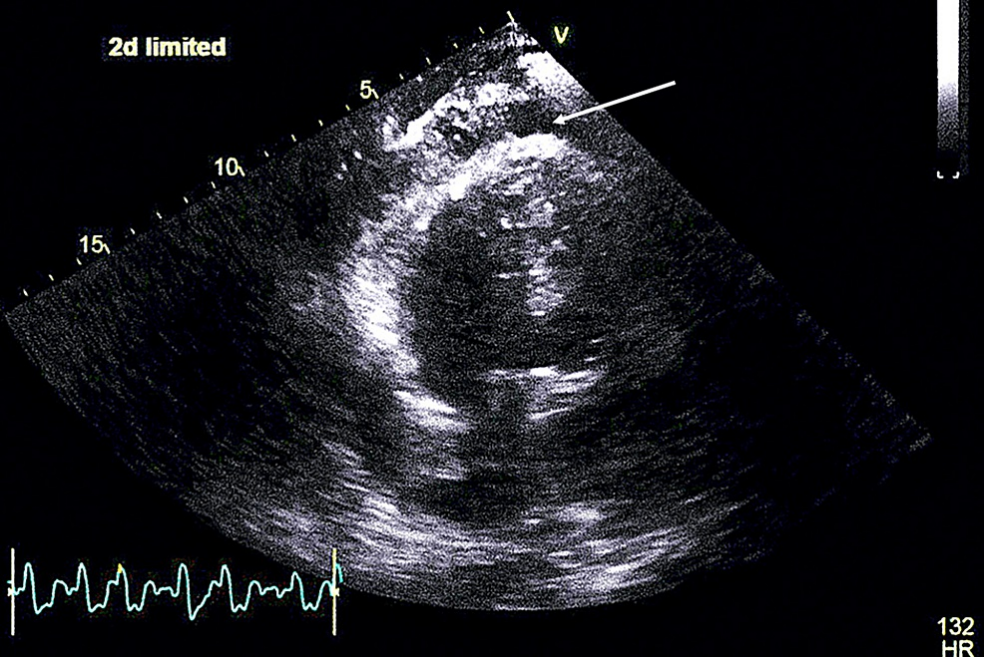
TEE showing moderate pericardial effusion. TEE, transesophageal echocardiography
